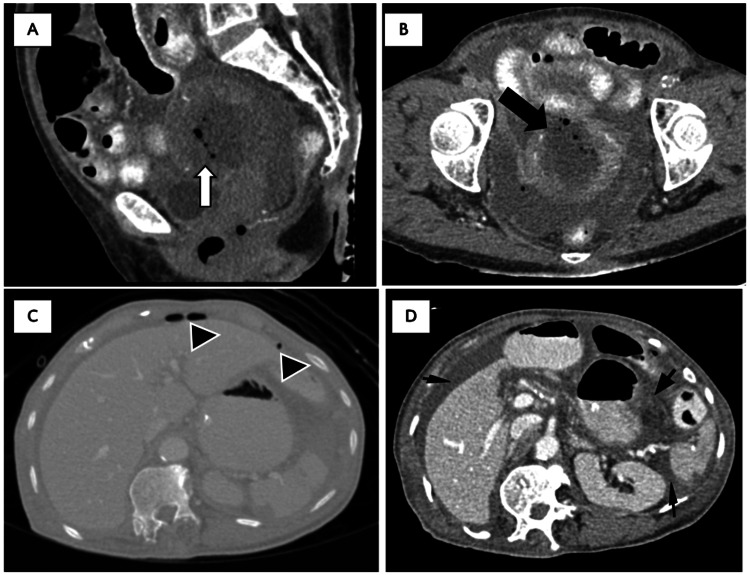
Contrasted CT images Sagittal reformatted CECT scan of the abdomen and pelvis showed an ill-defined hypodense collection (white arrow) within the uterine cavity with multiple air pockets (A), axial sections of the pelvis demonstrated focal uterine wall discontinuity (black arrow) at the fundus consistent with rupture (B), presence of pneumoperitoneum (arrowhead) (C) and intra-abdominal free-fluid (thin arrow) (D).
